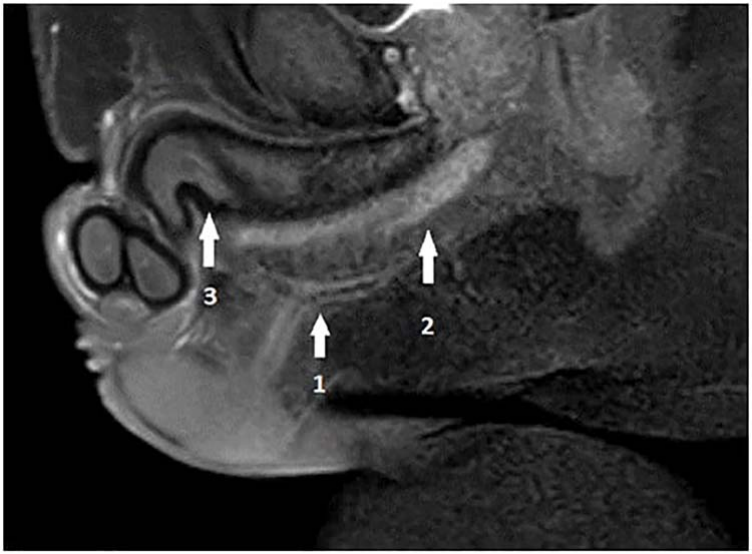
Pelvis MRI: horizontal one-eyed fistula (1) on the medial ligne above the corpus spongiosum (2), ending vertically with no collection and (3) corpus cavernosum.
